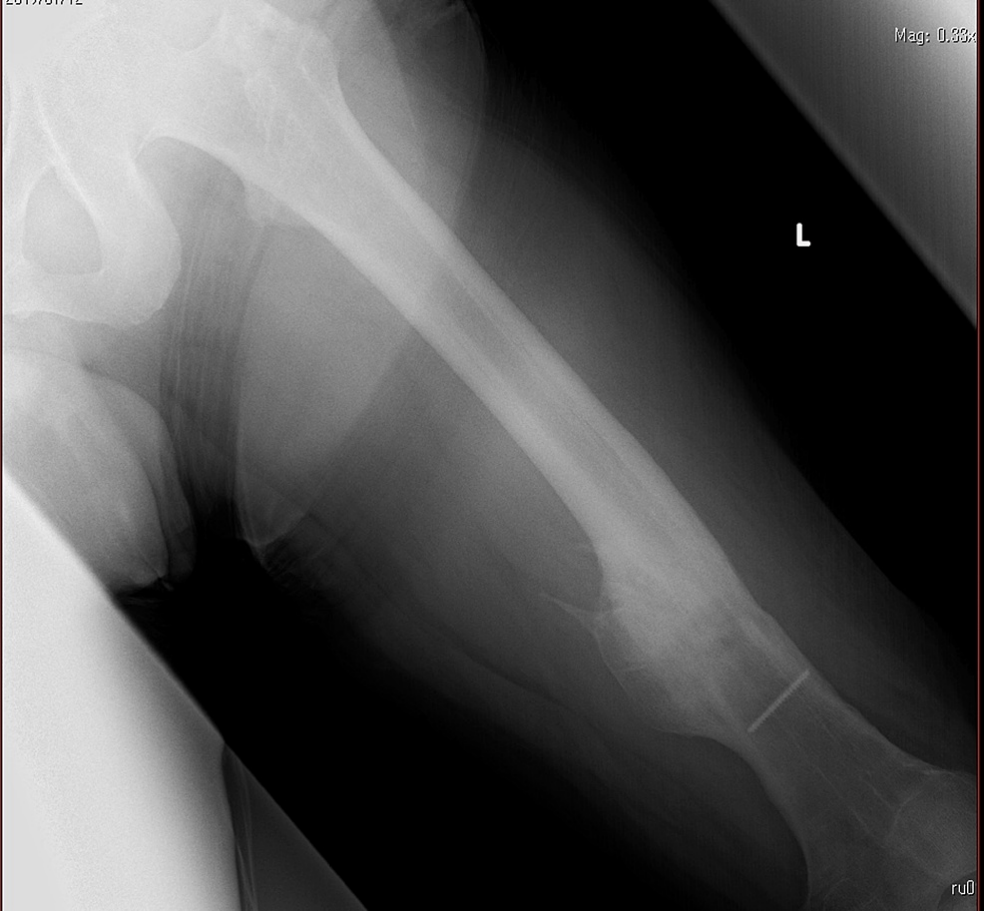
Left femur postoperative X-ray No abnormalities
